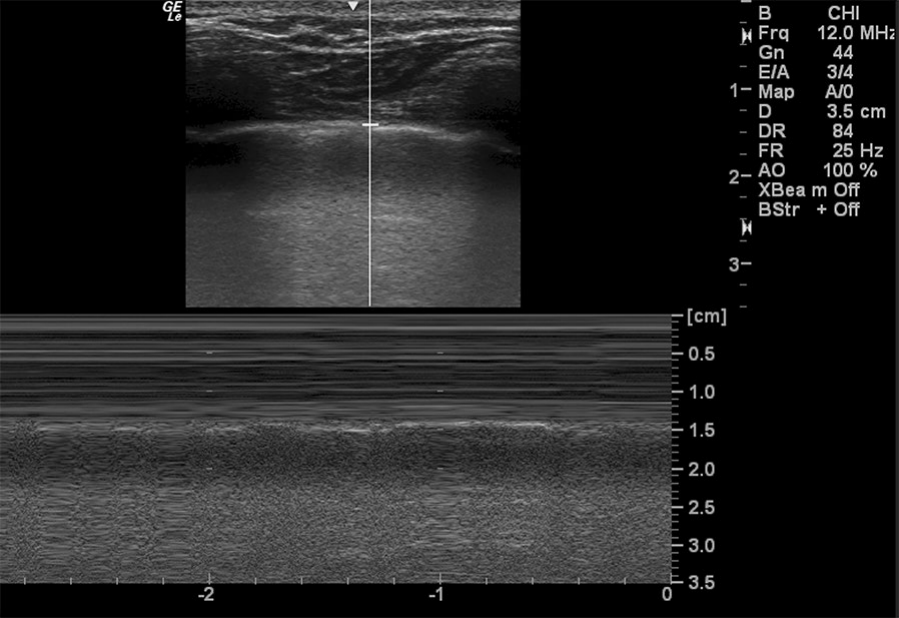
Parasternal view of the lungs in M-mode showing sea shore appearance that confirms absence of pneumothorax
